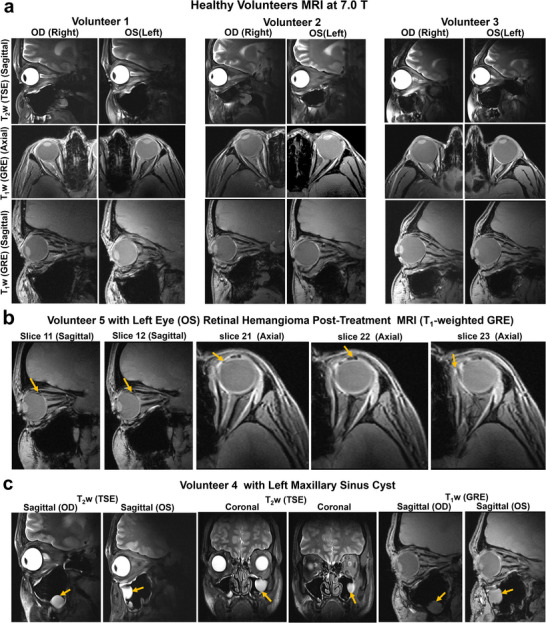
(a) High‐resolution anatomical 7.0 T MRI of three healthy volunteers (2 males, 1 female) using T_2_‐weighted Turbo Spin Echo (TSE, sagittal) and T_1_‐weighted Gradient Echo (GRE, axial/sagittal), acquired with the Bend‐MTMA configuration, enabling detailed visualization of the eye and orbital anatomy. (b) Clinical evaluation in a volunteer with prior retinal hemangioma pathology in the left eye, examined with MRI at 7.0 T using the Bend‐MTMA configuration 16 days post‐treatment. Sagittal and axial views of the eye and orbit reveal post‐treatment structural changes in the globe and anterior orbit, consistent with scarring and a periorbital vascular malformation (yellow arrow). (c) An incidental finding of a left‐sided maxillary sinus cyst (indicated by a yellow arrow) was identified in volunteer 4, appearing hyperintense on T_2_‐weighted MRI and visible on T_1_‐weighted MRI. The coronal slice demonstrates detailed visualization of the anterior cranial structures, including the extraocular muscles, optic nerve, and the cyst adjacent to the left orbit.
